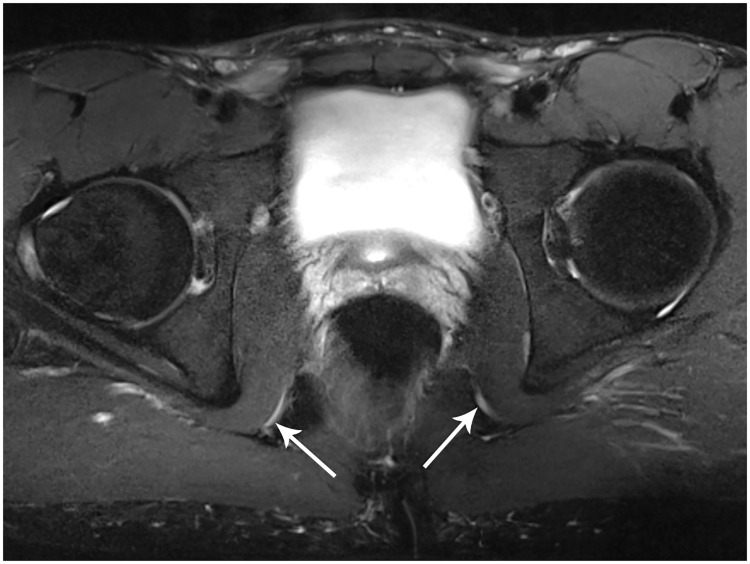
Pre-operative pudendal nerve 3T MR neurography. Axial high spatial resolution T2-weighted MR image with fat suppression demonstrate abnormally hyperintense and thickened pudendal nerves bilaterally (arrows).
